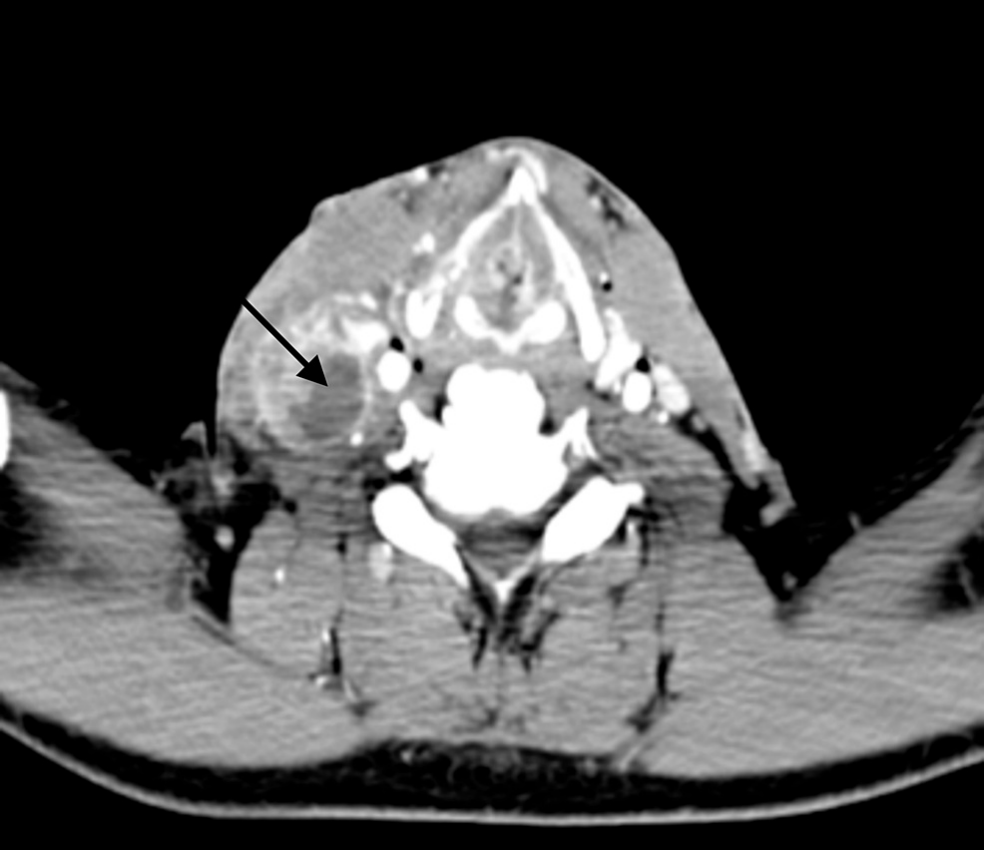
Computed tomography images of necrotic lymph nodes (necrotic lymph nodes in right level 3). Black arrow pointing towards the necrosis.
